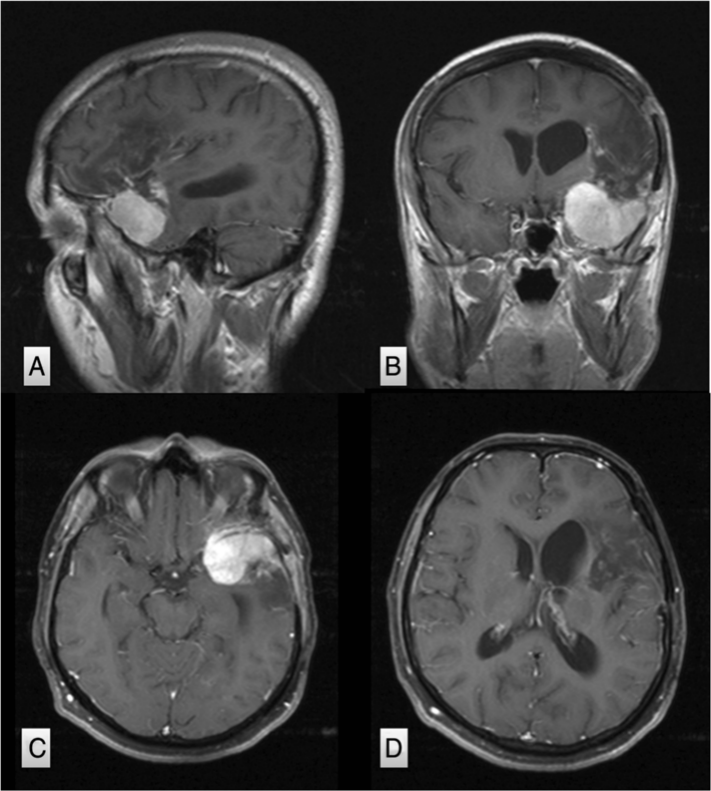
Post-contrast parasagittal (a), coronal (b), and axial (c, d) MRI scans performed 3 weeks after left frontal-temporal craniotomy demonstrating significant residual enhancing left sphenoid wing meningioma and ischemic changes in the left MCA territory
